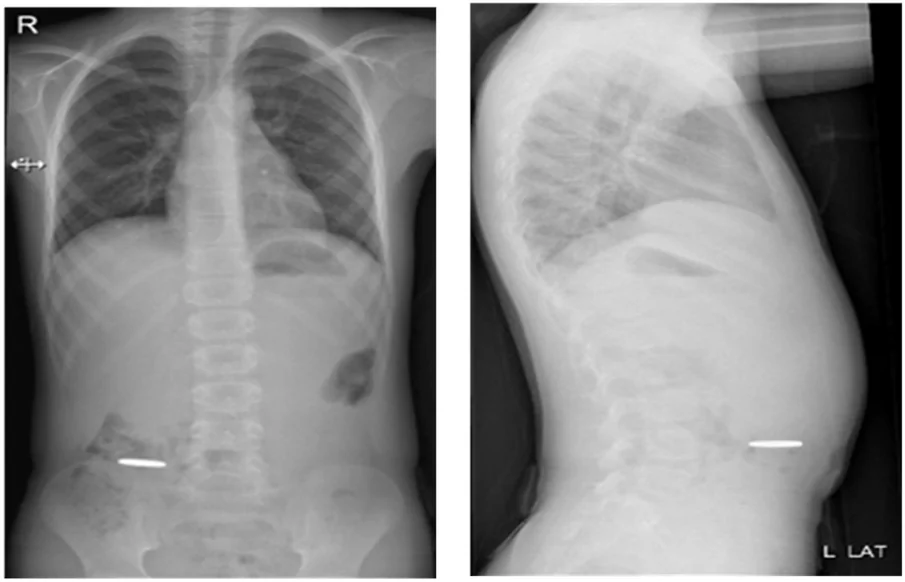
Follow-up posterior-anterior (A) and lateral (B) radiographs obtained one hour after the intervention showing the foreign body at the ilieo-cecal junction.
